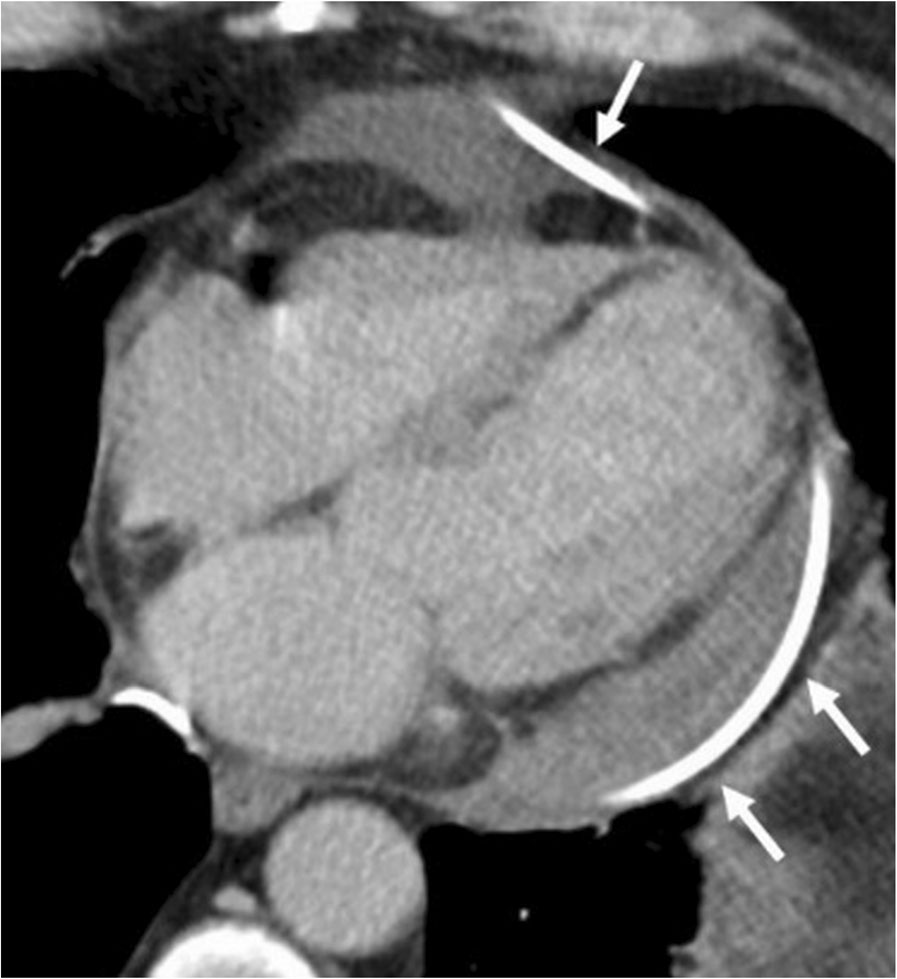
A 80-year-old man with echocardiographic findings suggestive of restrictive physiology due to pericardial effusion. Axial contrast-enhanced CT image demonstrates a drainage catheter coursing within pericardial space (arrows)
